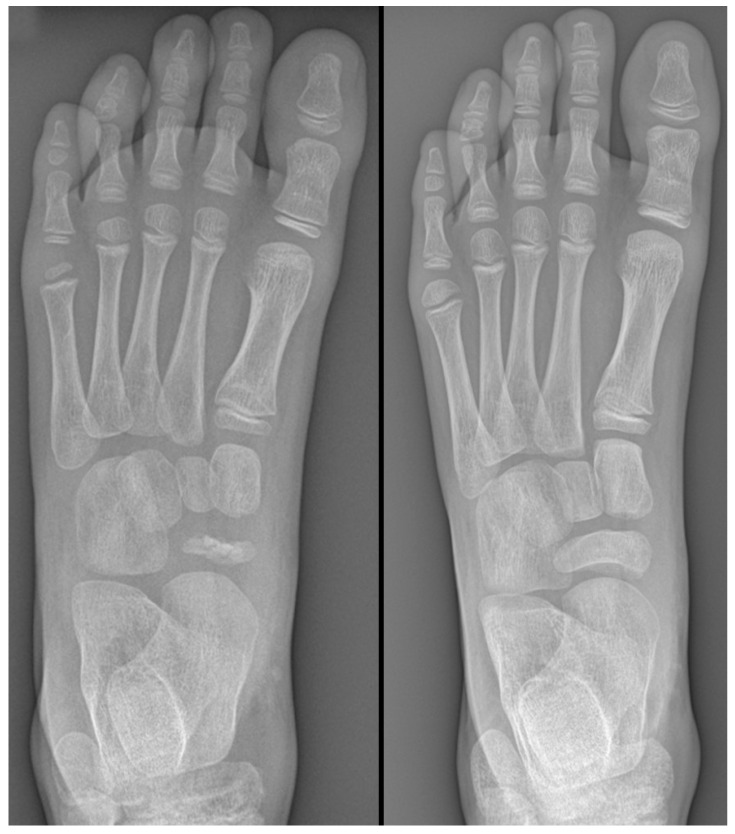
(Left): Radiograph of a 6-year-old male patient showing characteristic collapse and flattening of the navicular bone. (Right): Radiograph at 2-year follow-up after conservative treatment with non-weight-bearing casting, demonstrating good restoration of the navicular bone’s anatomical profile.
